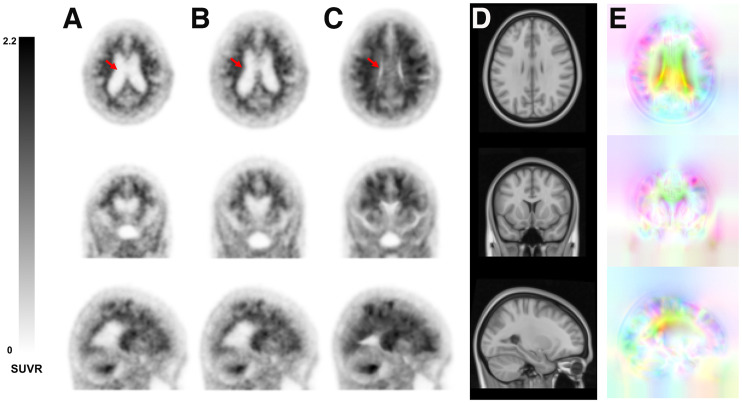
SN of ^18^F-florbetaben PET in amyloid-positive case: input image in individual space (A), MRI-based SN using SPM (B), PET SN using DNN (C), T1 MRI template (D), and estimated deformation fields using DNN (E). Red arrows indicate the enlarged ventricles, which are not properly deformed by SPM.
